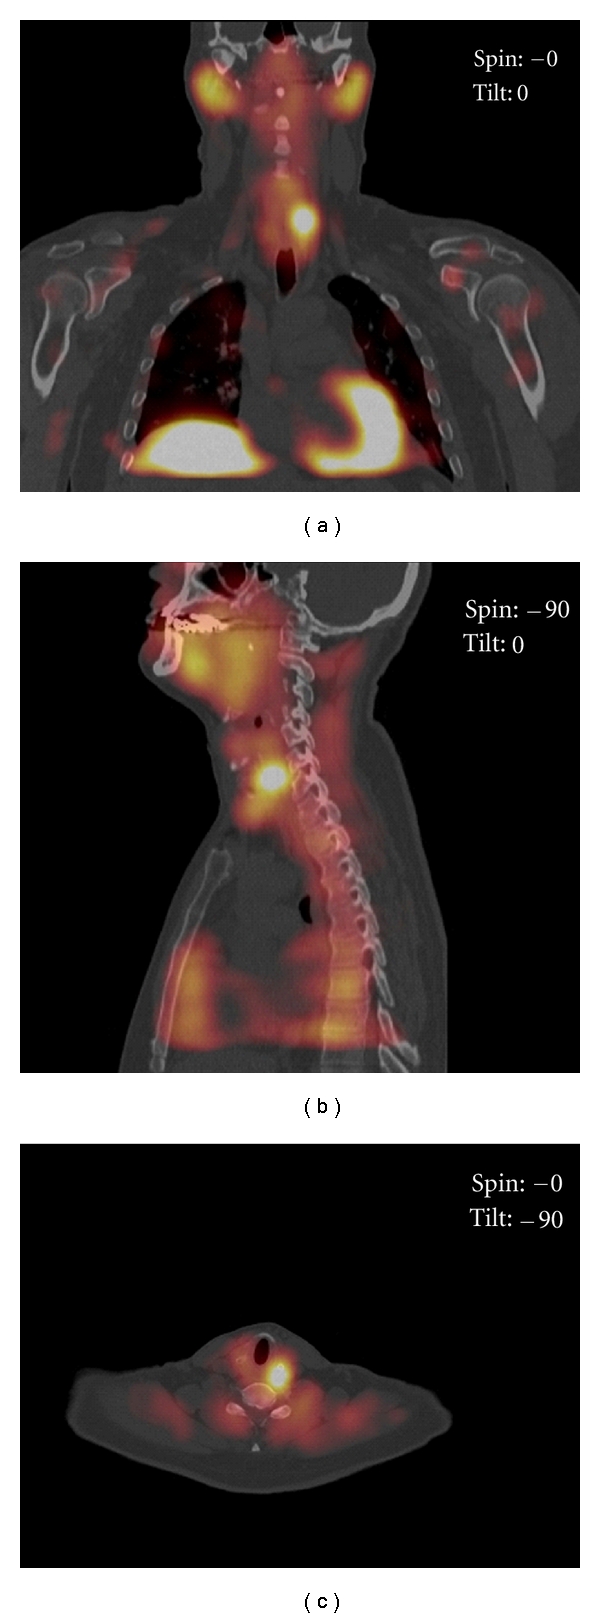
Left superior parathyroid adenoma with delayed washout. Delayed phase (a) coronal, (b) sagittal, and (c) transverse fused SPECT/CT tomograms show a left superior parathyroid adenoma with a posterior location at the upper pole of the left thyroid lobe.
